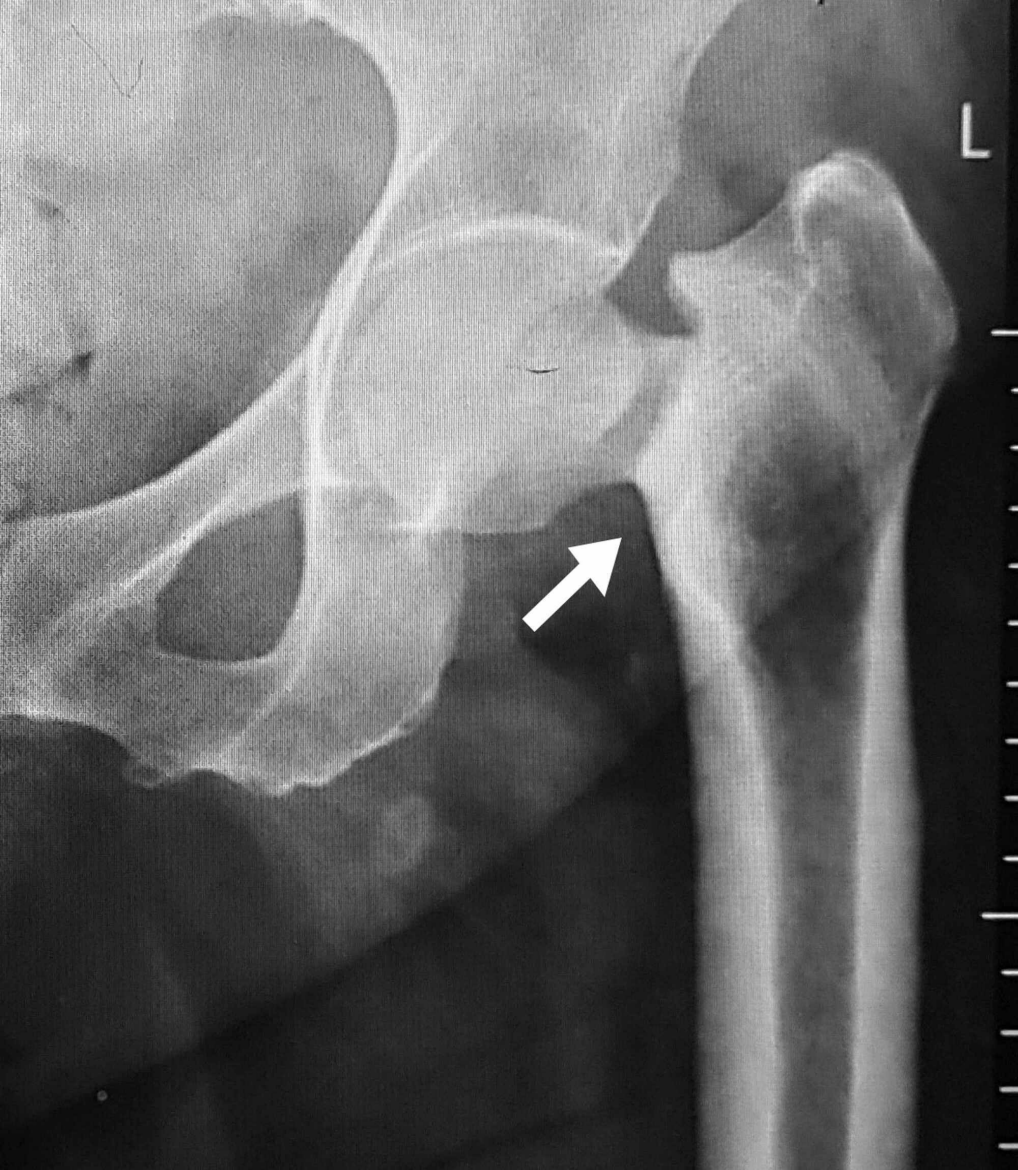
Anteroposterior left hip radiograph with displaced femoral neck fracture (white arrow).
